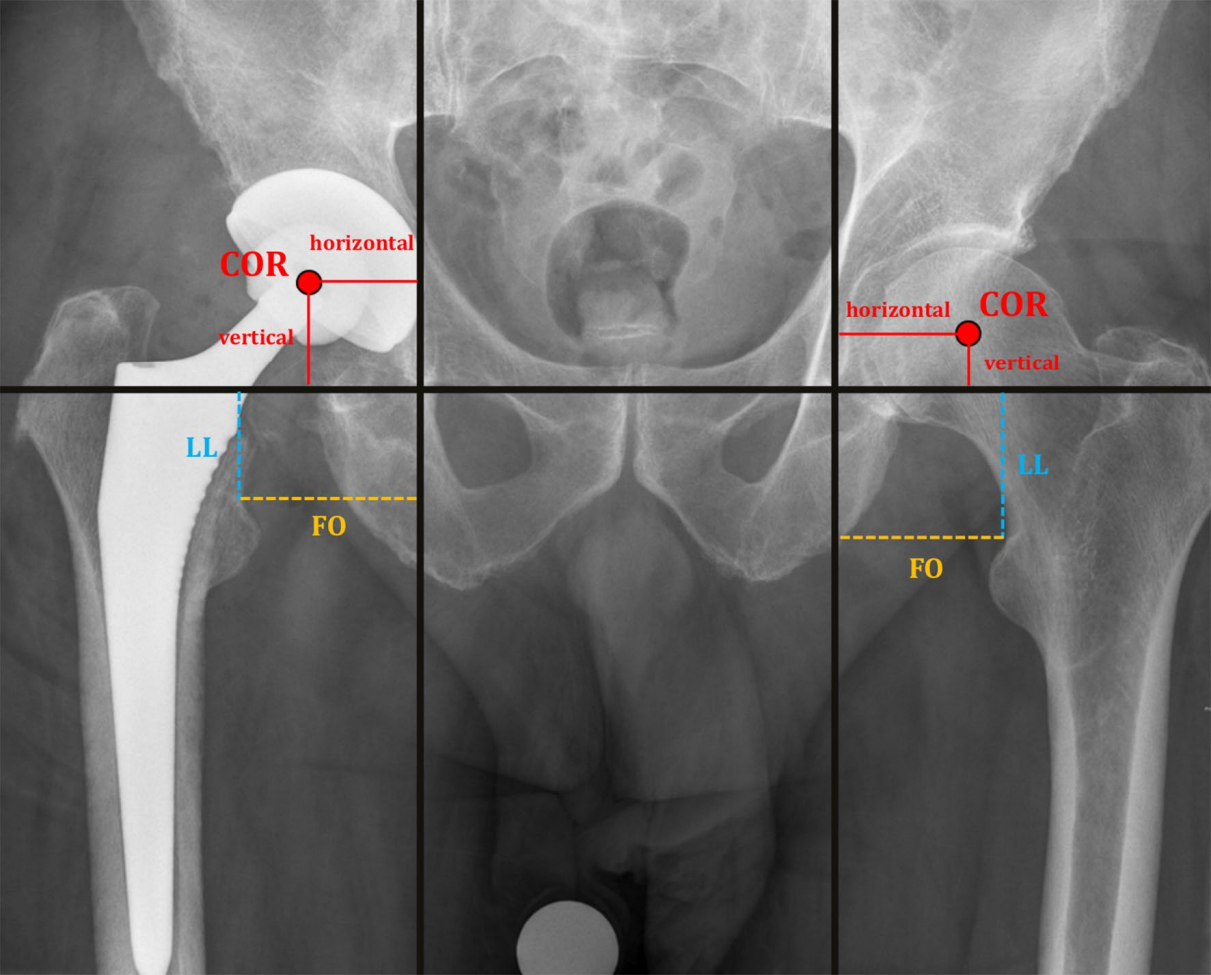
Standardized radiographs of the pelvis demonstrating the technique for measurement of the different radiographic parameters (leg length (LL), femoral offset (FO), horizontal and vertical acetabular center of rotation distance (hCORD, vCORD)) during PJI treatment [in mm]. LL (blue line) was measured as the length between the interteardrop line and the lesser trochanter. FO (yellow line) was measured as the length between the teardrop and the lesser trochanter. The vCORD and hCORD (red lines) were measured as the length between the teardrop and the center of rotation (COR).
